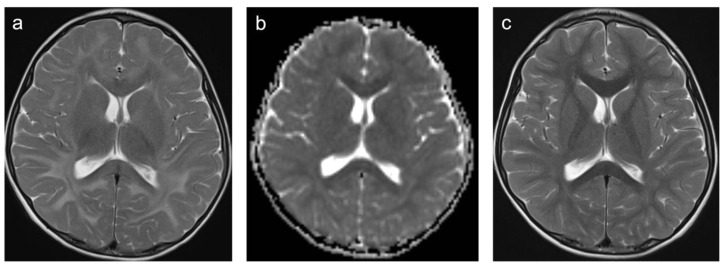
Representative case “Inborn errors of metabolism”: methionine adenosyltransferase I/III deficiency. T2W (a,c) sequences and ADC map (b). Three-year-old boy diagnosed with a methionine adenosyltransferase I/III deficiency. MRI shows T2W signal hyperintensities in subcortical and deep white matter with relative sparing of corticospinal tracts, corpus callosum and optic radiations (a). ADC map demonstrates decreased ADC value of the lesion (b). Normalization of established WMSAs within two years on a strict methionine-restricted diet (c).
